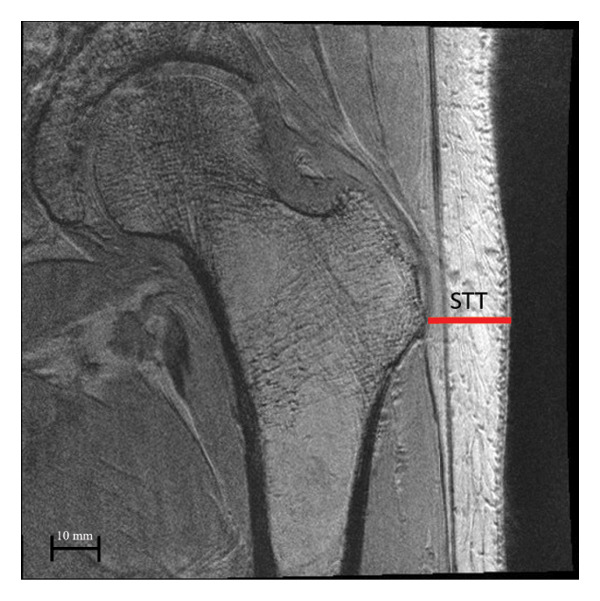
Derivation of trochanteric soft tissue thickness (STT) using patient MR images. STT was calculated as the distance between the greater trochanter and the skin.
